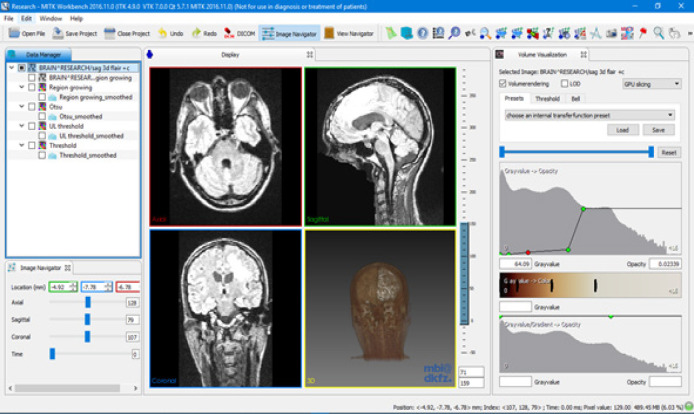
The User Interfaces in MITK Displaying the Orthogonal Slices of MRI in MPR Mode and the 3D View of the Volume in the Lower Right Quadrant
